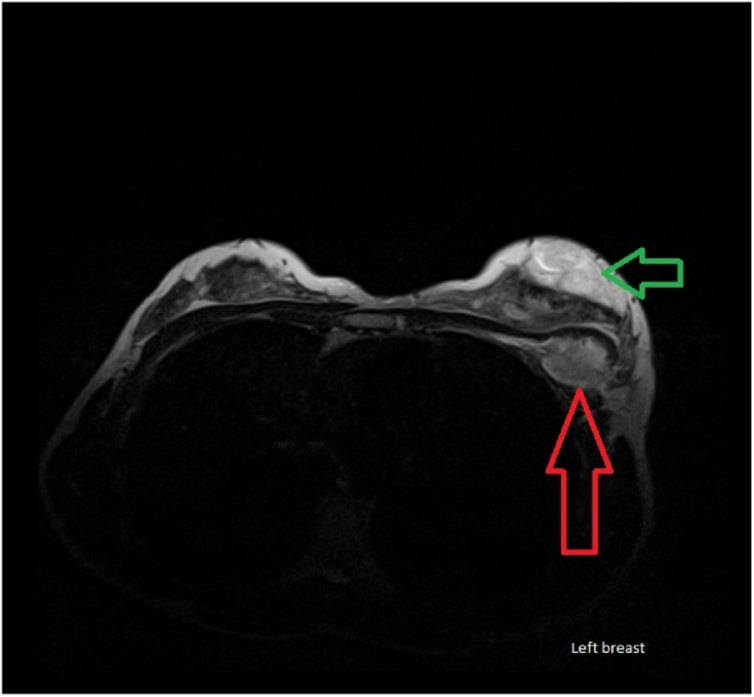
Breast MRI. Multifocal intra-parenchymal and sub-pectoral phyllodes tumor. Green arrow points towards intra-parenchymal Phyllodes tumor, red arrow points towards sub-pectoral Phyllodes tumor.
